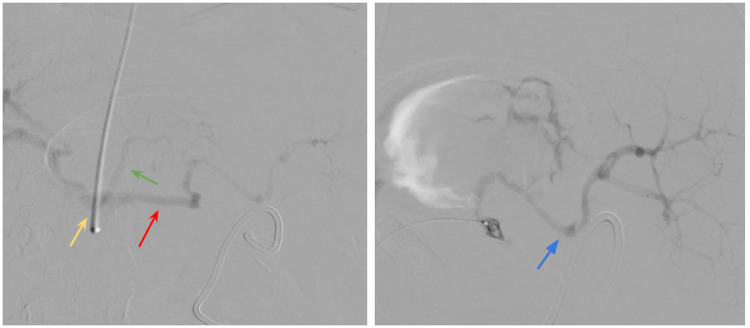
CT angiography. Catheterization of the celiac trunk (yellow arrow), left gastric artery (green arrow), splenic artery (red arrow), and splenic artery branch (blue arrow); no evidence of contrast extravasation.
